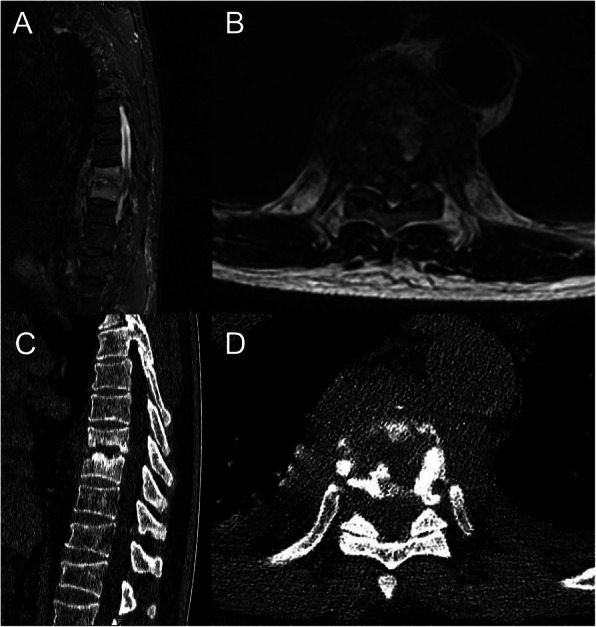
Radiological studies before operation. a Sagittal SPIR before operation demonstrated existence of abnormal signals in the intervertebral disk space between T9 and T10; b T2 weighted axial image showed well-defined abnormal paraspinal and intraspinal signals; c Sagittal CT image showed damaged vertebrae and endplate (T9/T10); d CT axial image showed paraspinal and intraspinal soft-tissue mass and partial osteosclerosis
